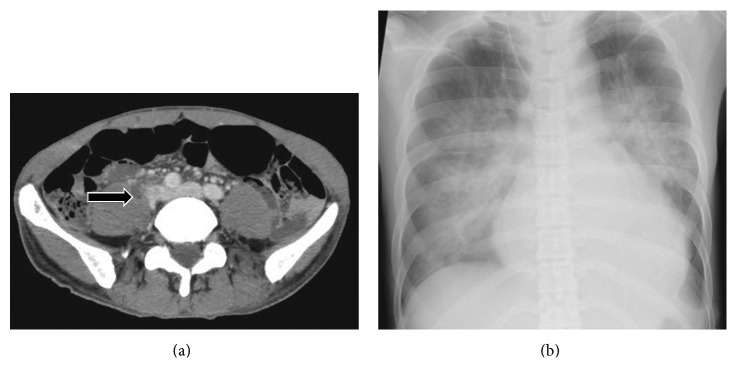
(a) There was a low-density foci with enhancement of the wall in the patient's right iliopsoas muscle, raising suspicion of iliopsoas abscess. (b) The result of chest radiography on day 3 showed severe cardiomegaly, measured as a cardiothoracic ratio of 61% with pulmonary edema.
